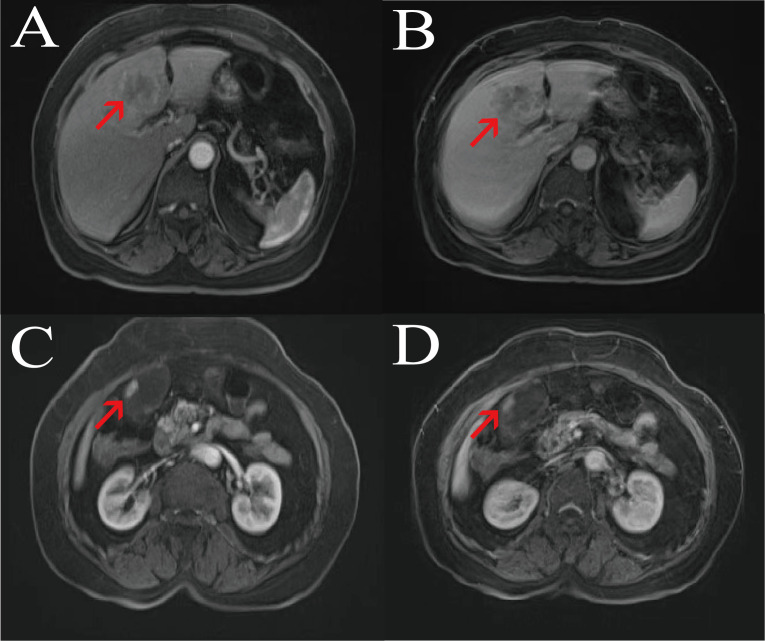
Contrast−enhanced MRI. (A) T1−weighted imaging of the liver in the arterial phase; (B) T1−weighted imaging of the liver in the portal venous phase; (C) T1−weighted imaging of the gallbladder in the arterial phase; (D) T1−weighted imaging of the gallbladder in the portal venous phase.
